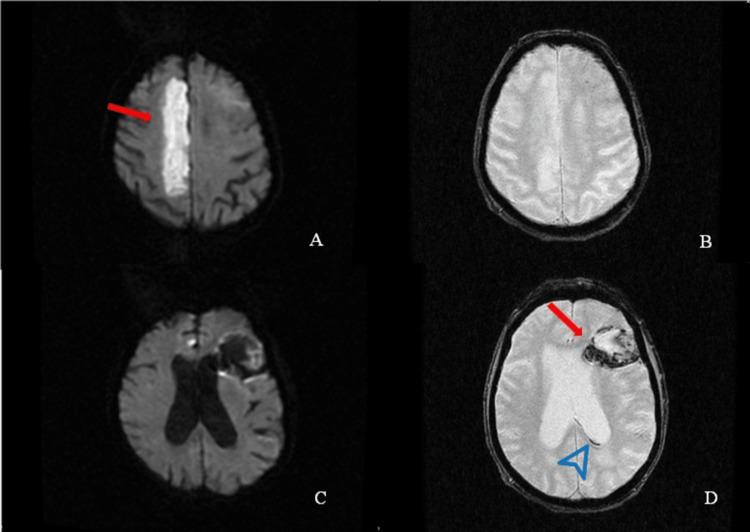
A brain MRI of the second patient with HBC-3 hemorrhagic conversion following tenecteplase administration Axial diffusion-weighted imaging (DWI) (A) and gradient recalled echo (GRE) MRI images of the brain at the level of the centrum semiovale demonstrate diffusion restriction (arrow) compatible with acute-subacute infarct in the right ACA territory with no hemorrhage in the corresponding territory on the GRE sequence. Axial DWI (C) and GRE (D) sequence images of the brain demonstrate left frontal middle cerebral artery (MCA) territory acute hemorrhagic infarct (arrow), with trace left intraventricular hemorrhage (arrowhead). HBC: Heidelberg bleeding classification
